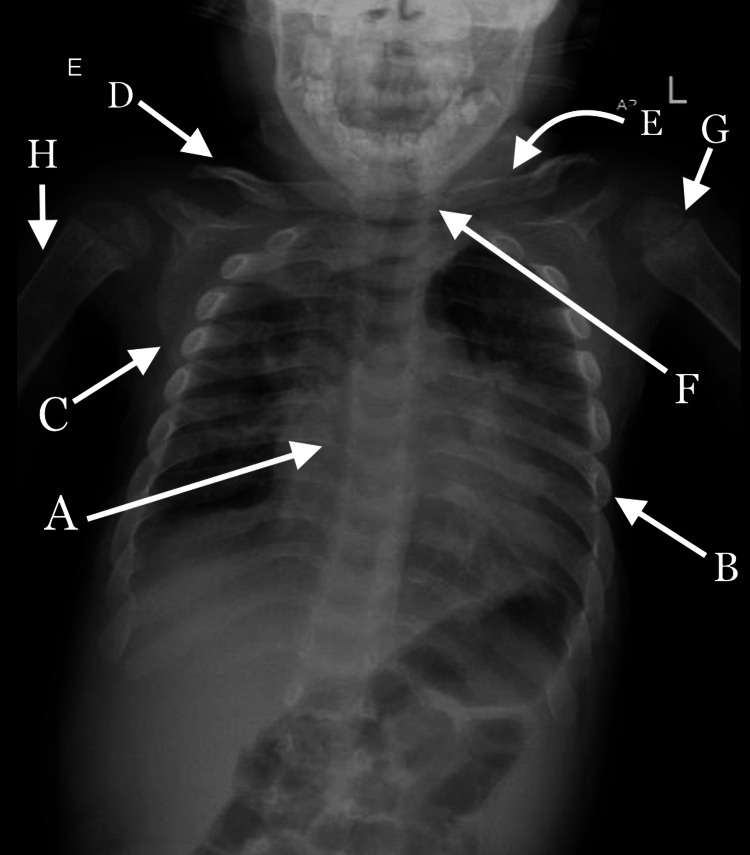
Chest X-ray Ribs narrowing on the sternum end [A], ribs widening on the vertebral end [B], dysplastic scapula [C], hypoplastic on the lateral end of the clavicle [D], broadening the clavicle [E], short neck [F], flattened epiphysis [G], wide short diaphysis [H]
